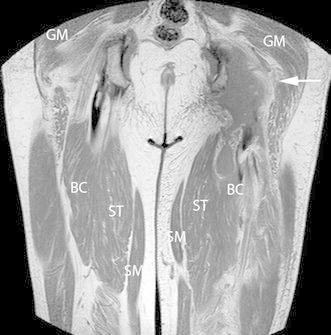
MRI of left hamstring avulsion (*GM* gluteus maximus, *BC* biceps femori, *ST* semitendinosus, *SM* semimembranosus)
